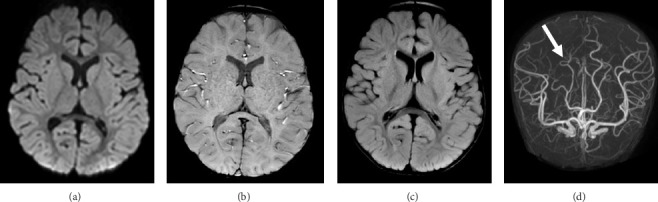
(a): An axial diffusion-weighted magnetic resonance (MR) image obtained at 16 days after stroke onset demonstrating the absence of an acute infarction. (b): An axial fluid-attenuated inversion recovery MR image depicting no radiological findings of infarction, even in the right parieto-occipital lobe. (c): An axial susceptibility-weighted MR image revealing no hemorrhagic lesion. (d): MR angiogram showing the recanalization of the occluded parieto-occipital artery.
